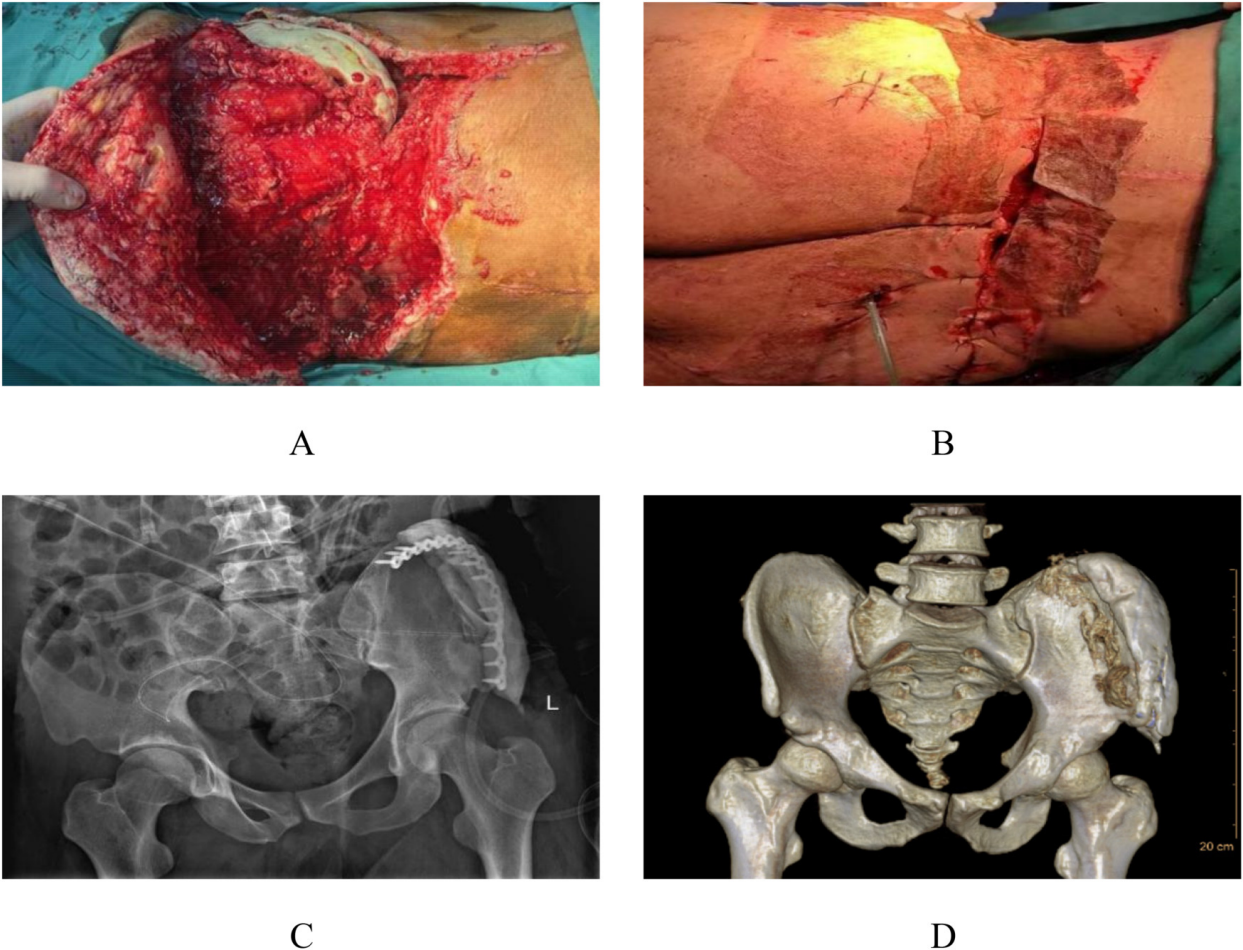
Intraoperative appearance during the second stage and postoperative imaging. The infected bone tissue was treated with antibiotic-loaded bone cement to address the bone infection (A), and continuous negative pressure drainage was continued to reduce infection (B) A follow-up x-ray was performed 1 week postoperatively (C) Follow-up CT scans were conducted 1 week after the operation (D).
